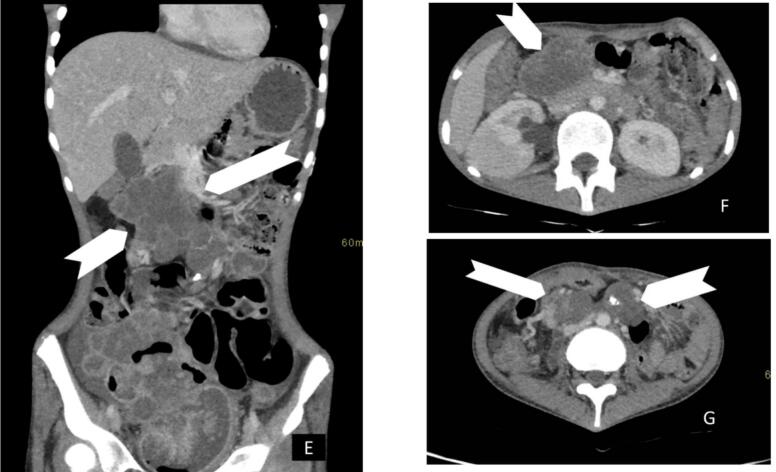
Computed tomography Images, (E) coronal, (F) axial images at the level of the kidneys, and (G) axial image at the level of the umbilicus demonstrating enlarged lymph nodes with calcification (white chevron).
